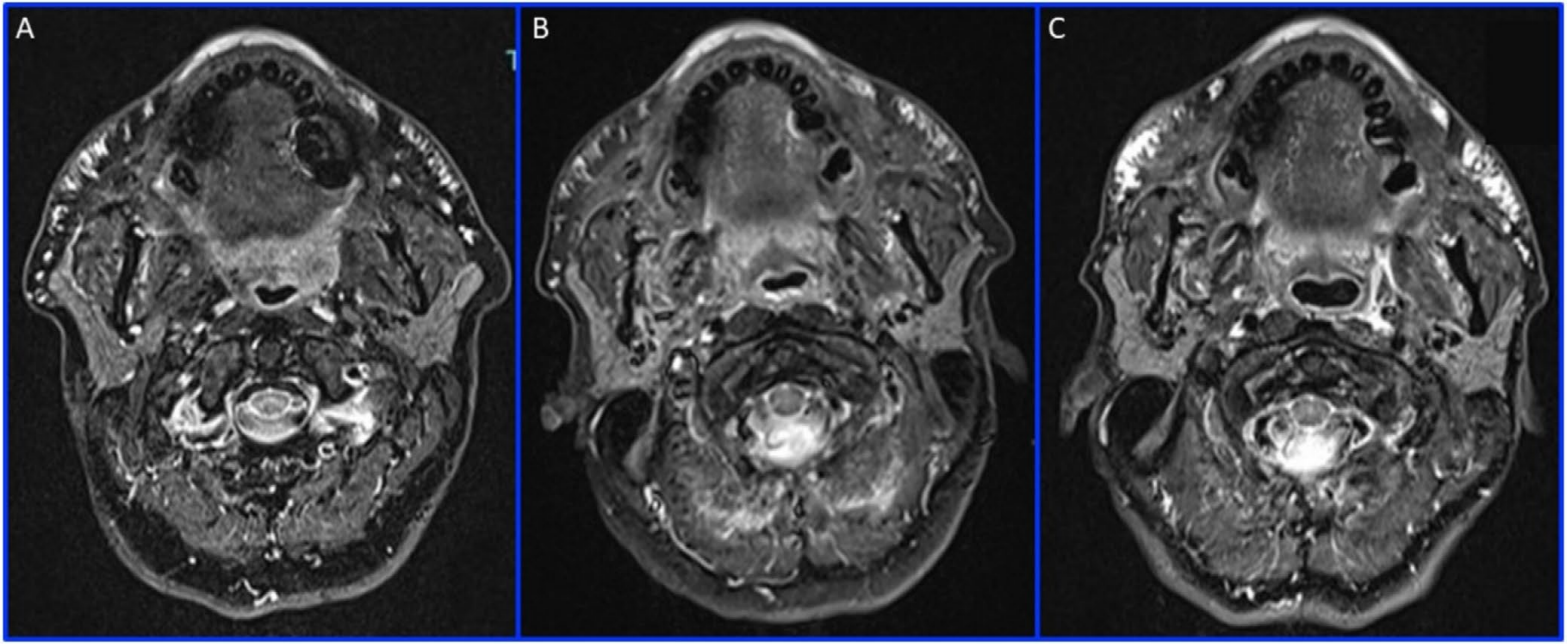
MRI: Axial T2 TIRM in I (A), II (B), and III (C) MRI, showing the persistence of HA filler in the upper lip, injected using a threading technique.
